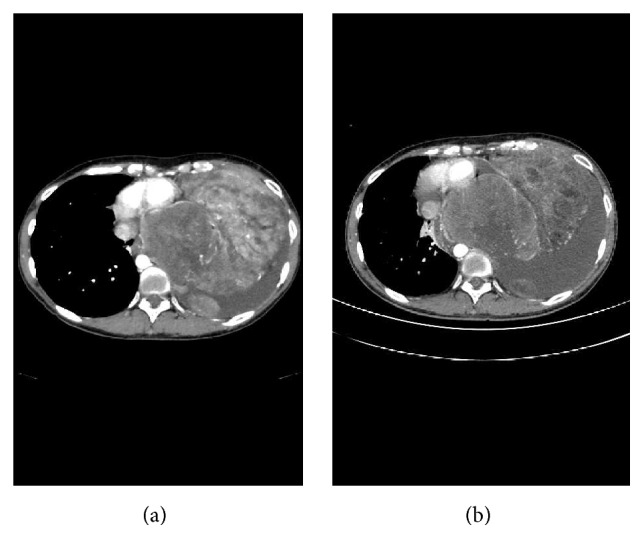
(a) Chest contrast-enhanced computed tomography (CT) before treatment showing a bulky mass in the left chest; pleural dissemination is seen. (b) Chest contrast-enhanced CT after 2 cycles of chemotherapy with doxorubicin showing reduction of the tumor.
